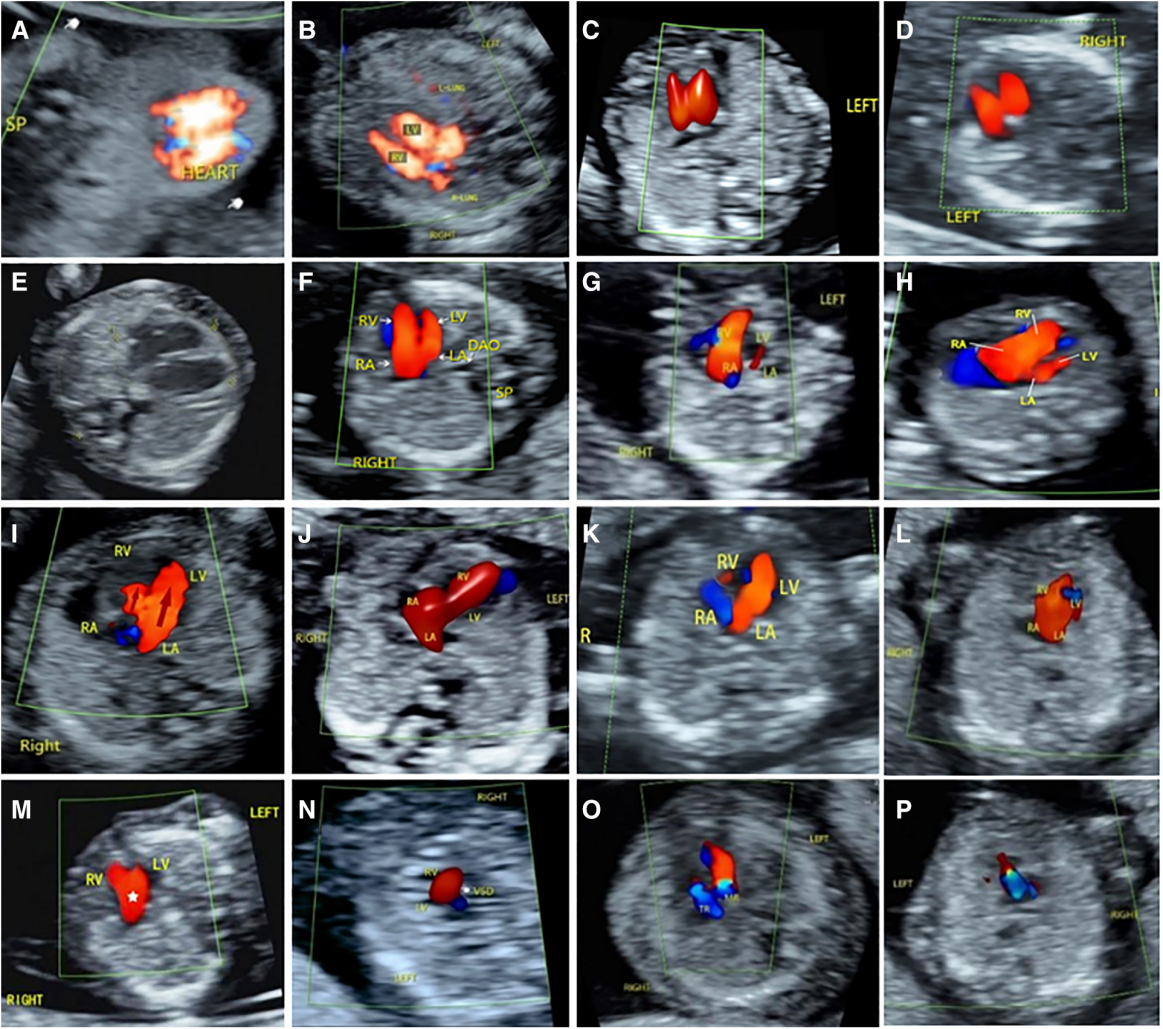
Abnormal ultrasound image pattern of 4CV section. (A) Patterns of 4CV-1 showed that the heart is partially or completely located outside the chest cavity. (B) Patterns of 4CV-2 showed displacement of heart. (C) Patterns of 4CV-3: the cardiac axis is abnormally shifted to the forward. (D) Patterns of 4CV-4: The cardiac axis is abnormally shifted to the right. (E) Patterns of 4CV-5 indicate an increase in cardiothoracic ratio. (F) Patterns of 4CV-6, filling of both ventricles, but the left ventricle appears smaller. (G) Patterns of 4CV-7: The left heart is spherically enlarged, and the filling of left ventricle appears smaller. (H) Patterns of 4CV-8: The filling of the right heart increased obviously. (I) Patterns of 4CV-9, filling of both ventricles, but the right ventricle appears very smaller. (J) Patterns of 4CV-10, filling of only the right ventricle. (K) Patterns of 4CV-11, filling of only the left ventricle. (L) Patterns of 4CV-12 showed a central singular inflow. (M) Patterns of 4CV-13 indicate “Y”-shaped filling of the ventricular inflow tract during diastole. (N) Patterns of 4CV-14 indicate a ventricular septal defect. (O) Patterns of 4CV-15, mitral or tricuspid regurgitation. (P) Patterns of 4CV-16, common valve regurgitation in atrioventricular septal defect.
